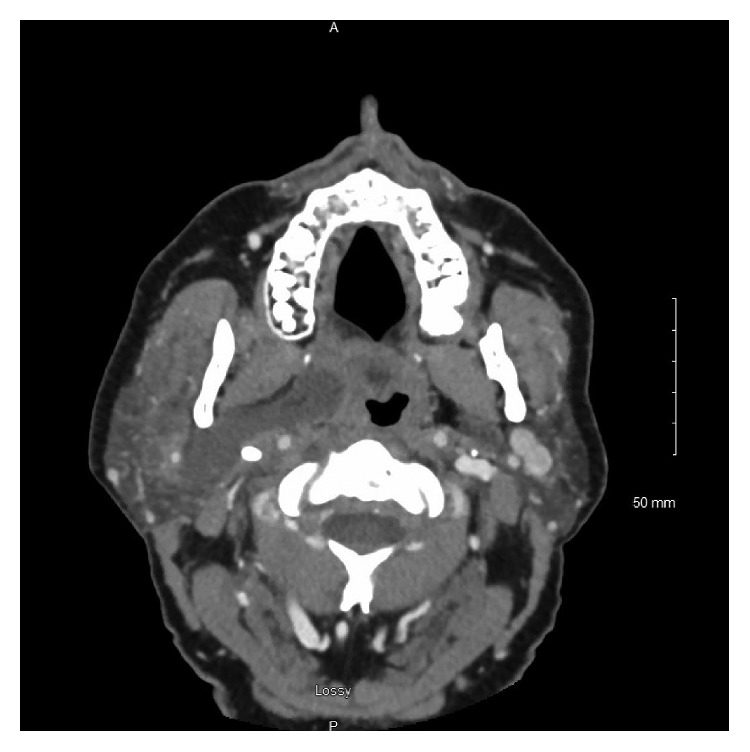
Axial CT image revealing retromandibular right deep lobe parotid abscess.
